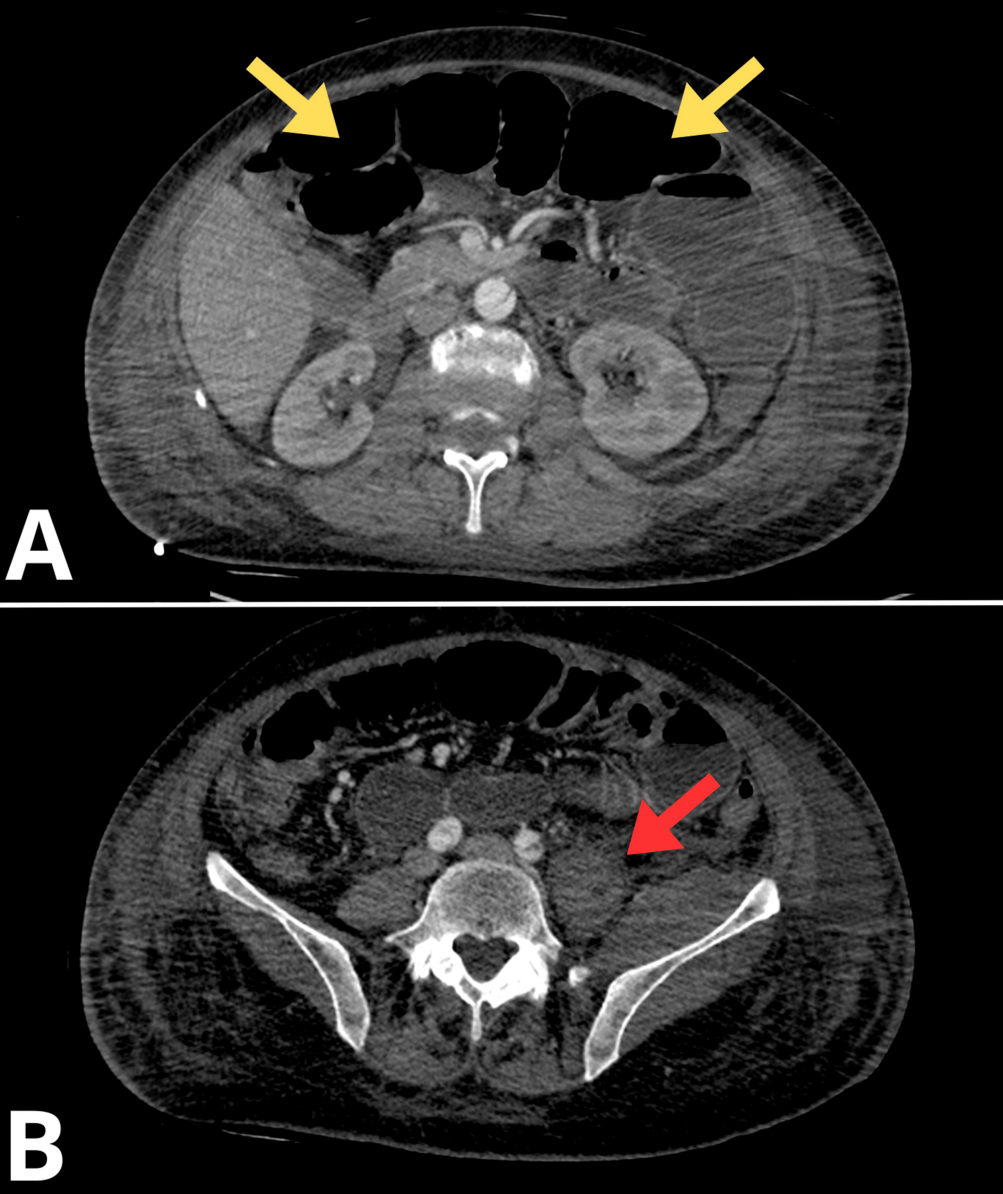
Angio-CT Transverse Scan of the Abdomen (A) Yellow arrows indicate dilated intestinal loops. (B) The red arrow indicates the swollen left iliopsoas muscle CT: computed tomography
